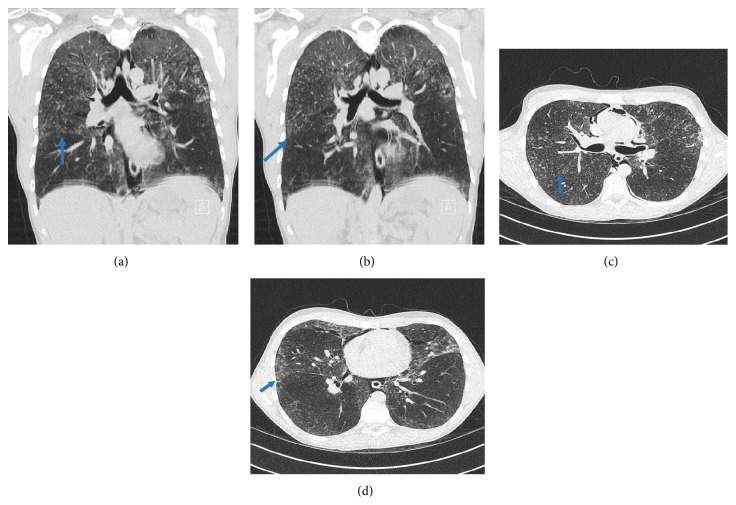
CT scan. (a)-(b) Coronal sections showing the bifurcation of the bronchus to the apical segment and the basal segments. There is no evidence of accessory branching, so no morphological extra lobe; hence there is only an accessory fissure (arrows). CT scans (a) and (b) have respiratory artifacts as seen at the level of the diaphragm. (c)-(d) Transversal sections showing the superior accessory fissure of the lower lobe of the right lung.
